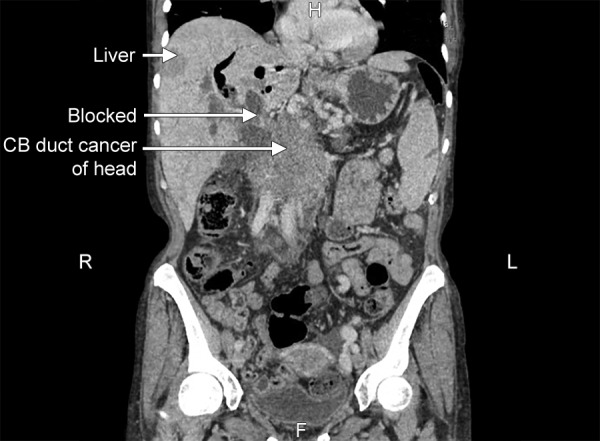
Computed tomography scan of abdomen showing pancreatic cancer with metastasis
